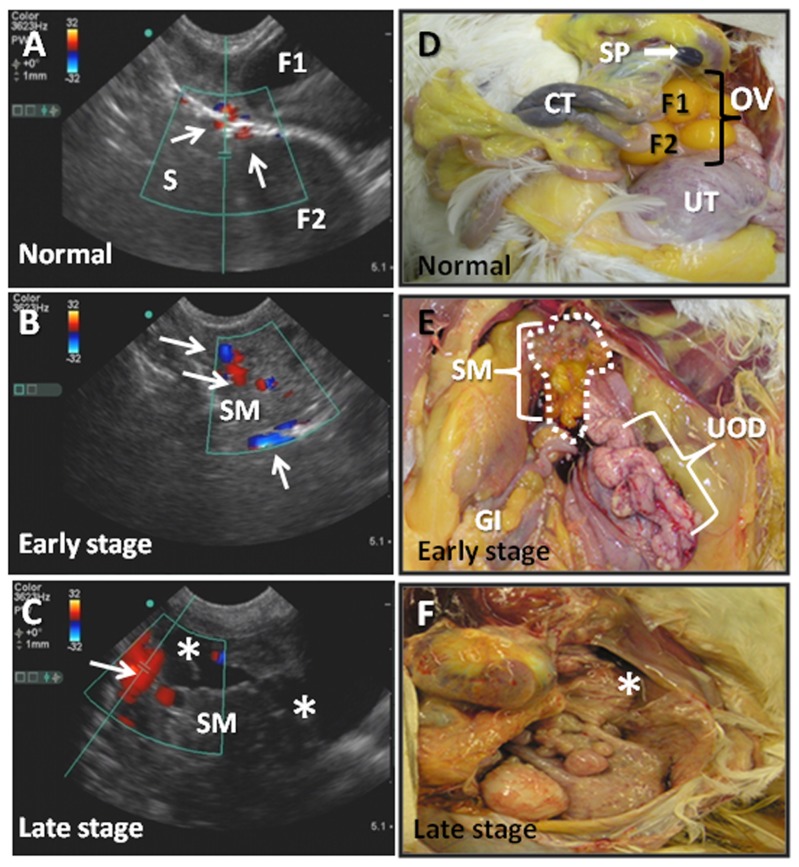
Selection of hens with normal ovaries or ovarian tumors using (A–C) color Doppler ultrasound and (D–F) gross morphology of the ovaries. A normal ovary (A and D) with maturing ovarian follicles (F1, F2) and stromal blood vessels (arrows) with normal gross morphology showing maturing (F1, F2) ovarian follicles. An example of an abnormal ovary (B and E) that contains more blood vessels (arrows) than in the normal ovary, no detectable large mature follicles and a small solid tissue mass (circle) in the ovary. An example of late stage ovarian cancer (C) characterized by a large solid mass with increased blood flow (arrows) and profuse ascites (*).The gross morphology (F) confirmed the presence of multiple solid masses and tumor metastasis to other organs. Abbreviations: CT = cecal tonsil; F1–F2 = large preovulatory follicles; GI = gastrointestinal tract; OV = ovary; S = ovarian stroma; SM = solid tissue mass in the ovary; SP = spleen; UOD = upper oviduct; UT = uterus.
